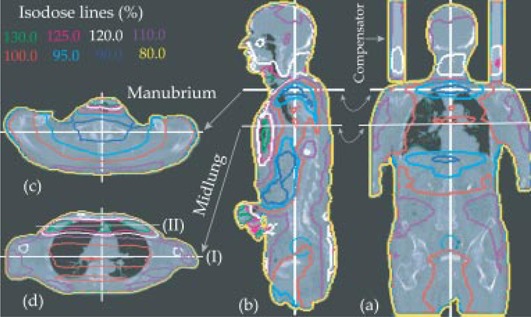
Isodose distribution shown on a patient CT, as calculated by treatment‐planning system for lateral‐opposed beam. The horizontal white lines show the levels of the transverse images (manubrium and midlung) to the left of the sagittal images. (a) coronal; (b) sagittal; (c) transverse manubrium; (d) transverse midlung image.
